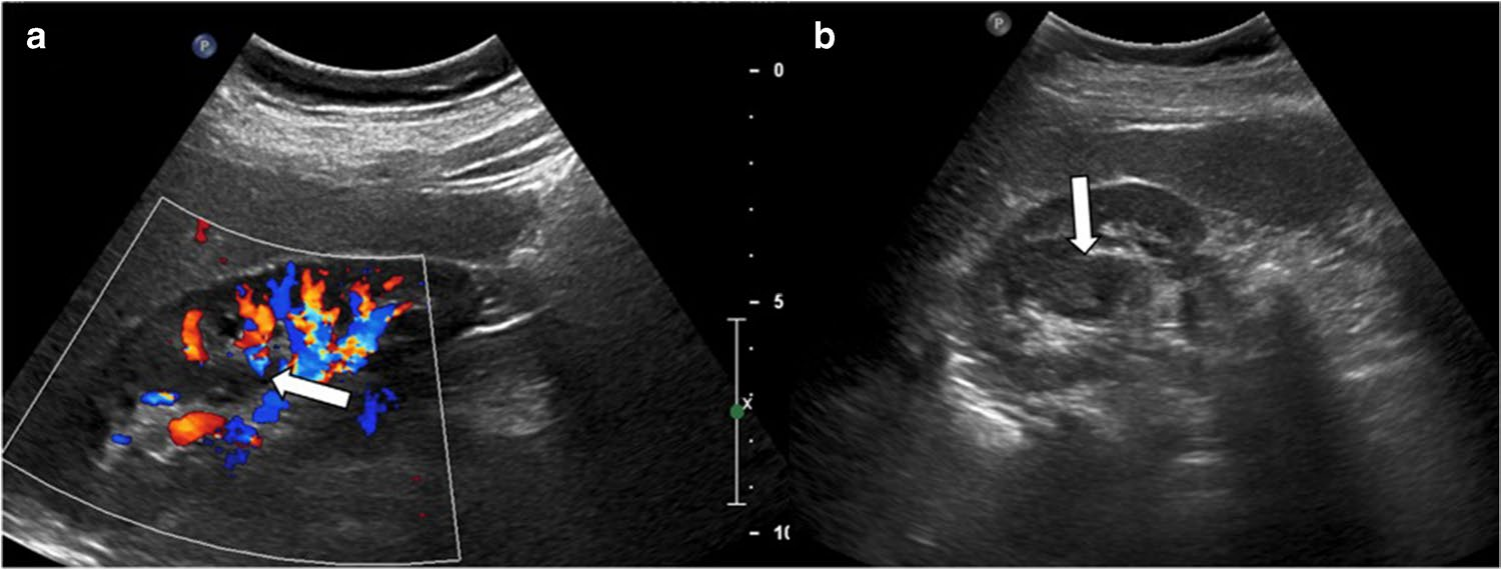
Prominent column of Bertin. A 13-year-old boy presented to the Emergency Department with abdominal pain. a Longitudinal color Doppler ultrasound (US) image shows that there is no abnormal internal vascularity (*arrow*) in contrast to the renal tumors. b Axial grayscale US image demonstrates a hypertrophied column of Bertin (*arrow*) in the transition between the upper and the middle thirds of the right kidney. Note that this area is homogeneous and has a similar echotexture to the surrounding parenchyma, and the renal contour is preserved
